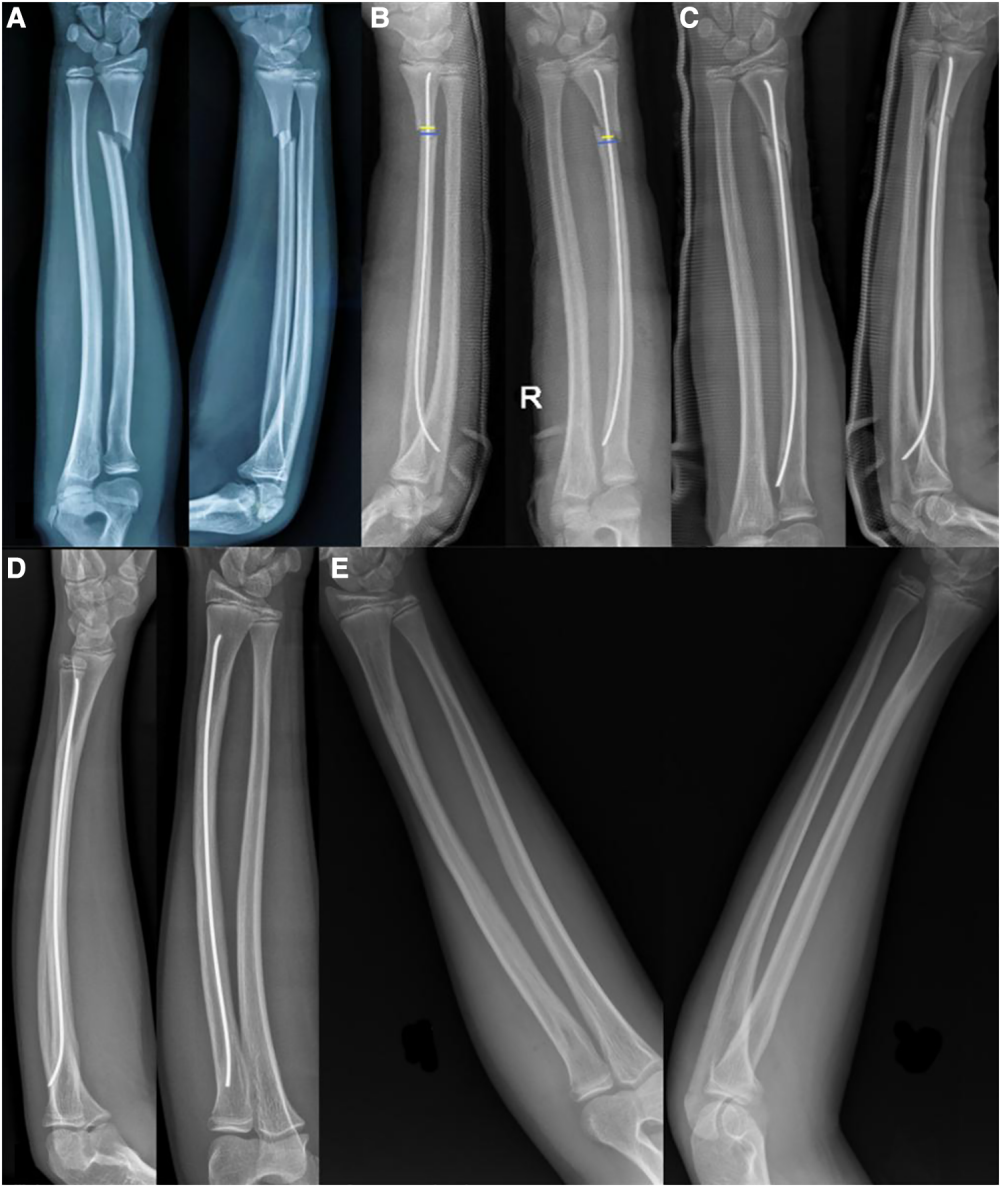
(A) An 11-year-old boy with obvious displacement of a right DRMDJ fracture on AP and lateral x-ray films; (B) after ESIN-A fixation, the alignment of the fracture was not good and showed residual radial displacement (yellow line was the apposition length of both fragments, blue line was the length of fracture line, and yellow/blue line was the alignment rate). (C) Two months after the operation, callus formation was showed, and residual lateral displacement and dorsal angulation were observed. (D) One-and-a-half years after the operation, the fracture was completely healed, and the residual dorsal angulation still exists. (E) The ESIN was removed, and the fracture completely healed.
